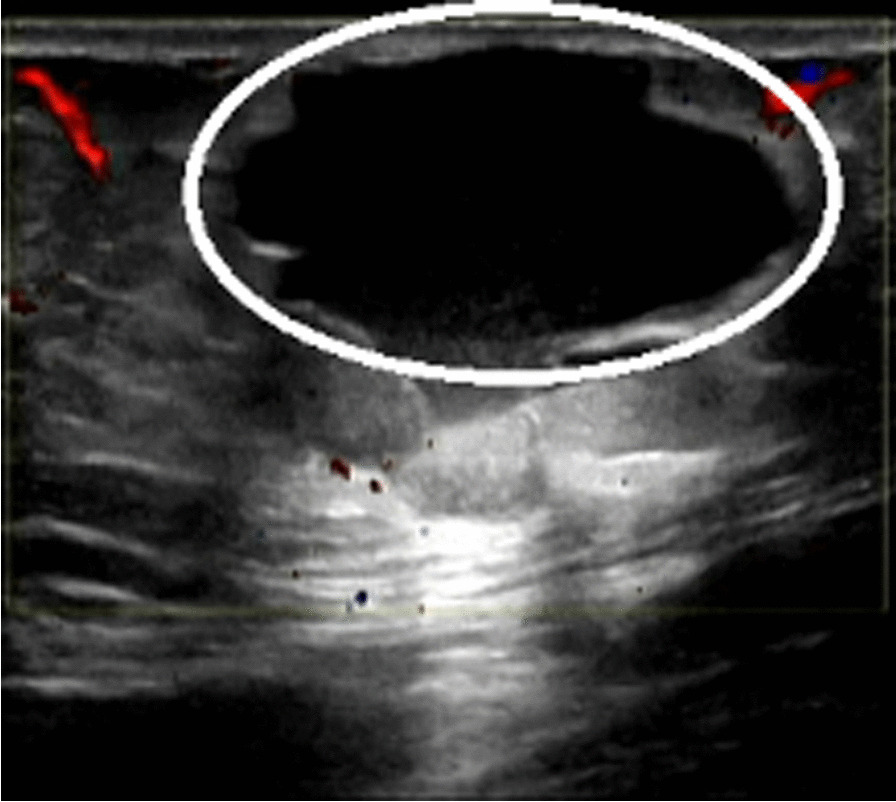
Ultrasound image showed cystic mass in subcutaneous fat layer of right lower abdomen (3.1 × 2.3 cm)
